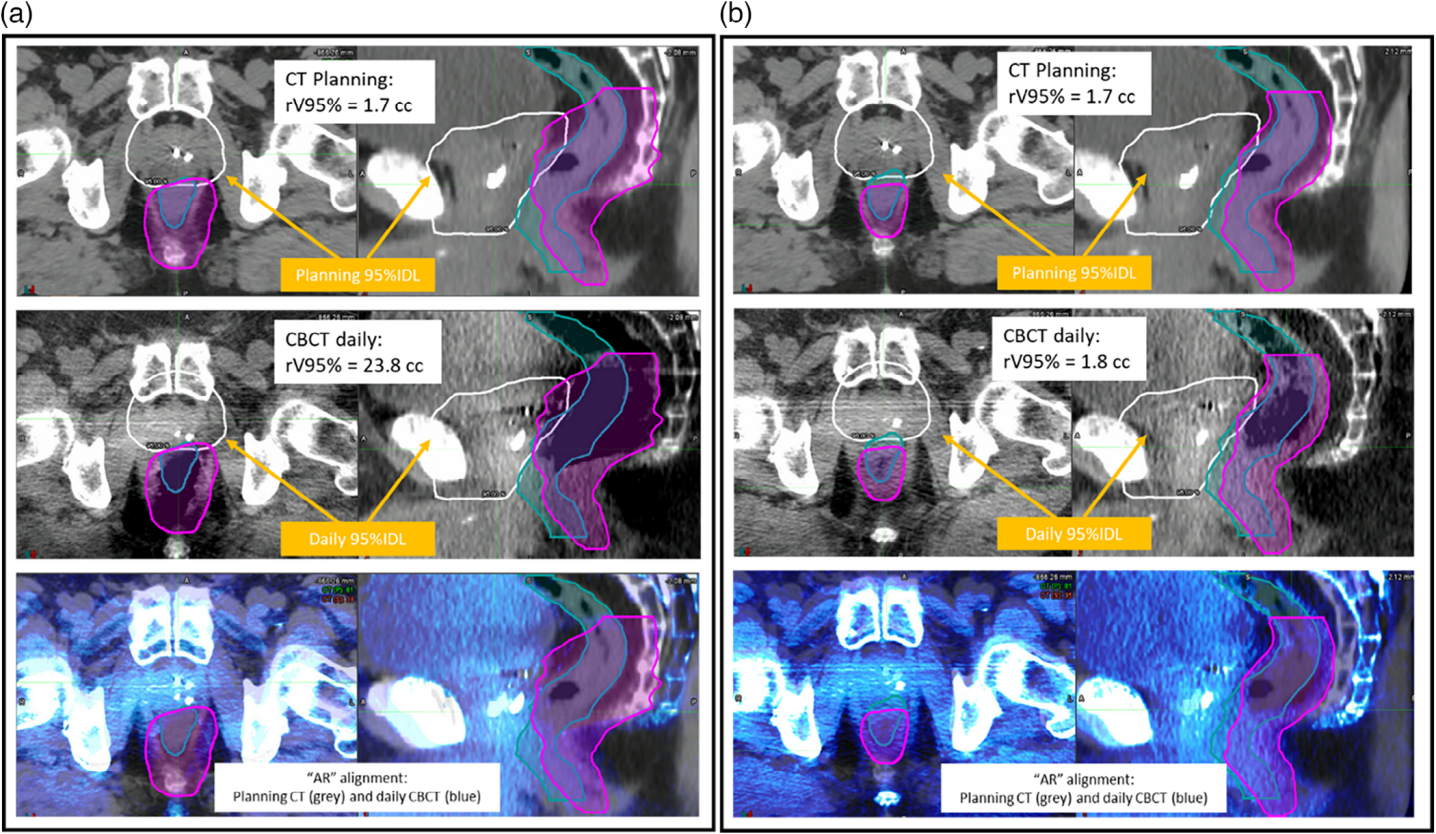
Daily (pink) and planning (teal) rectum contours and the corresponding rV95% IDLs (white). (a): Example of daily scenario where “AR” rV95% exceeds the planning value (23.8 cc vs. 1.7 cc). (b): Example of daily scenario where “AR” rV95% is similar to the planning value (1.8 cc vs. 1.7 cc). Top row: Planning CT and corresponding V95% IDL. Middle row: Daily CBCT and the corresponding V95% IDL. Bottom row: “AR” fusing of the planning CT and the daily CBCT. CBCT, cone beam computed tomography.
